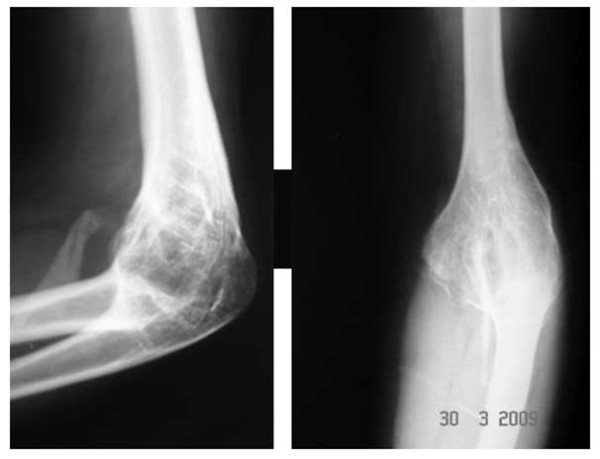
Preoperative lateral and antero-posterior radiograph of 28 year Female demonstrating bony ankylosed elbow with matured HO anteriorly.
